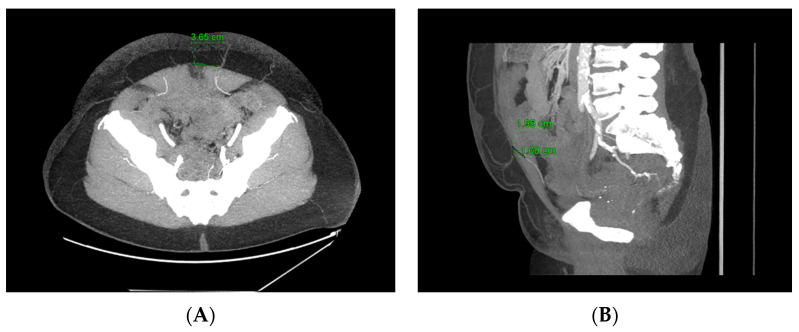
Computed tomography angiography (CTA) scan indicating a good candidate for a robotic-assisted deep inferior epigastric perforator (DIEP) flap due to the single dominant perforating vessel’s short intramuscular course (<3 cm long). The left deep inferior epigastric artery (DIEA) has a type 1 branching pattern. The perforator penetrates the fascia 6.0 cm below umbilicus reference point and 3.7 cm to the left (A). The subcutaneous segment courses anteriorly and branches throughout the subcutaneous fat. The subfascial segment is 1.1 cm long, coursing caudal (B). The intramuscular segment is 2.0 cm long, coursing caudal and lateral deep to muscle to join the main trunk, which courses 7.4 cm caudally.
